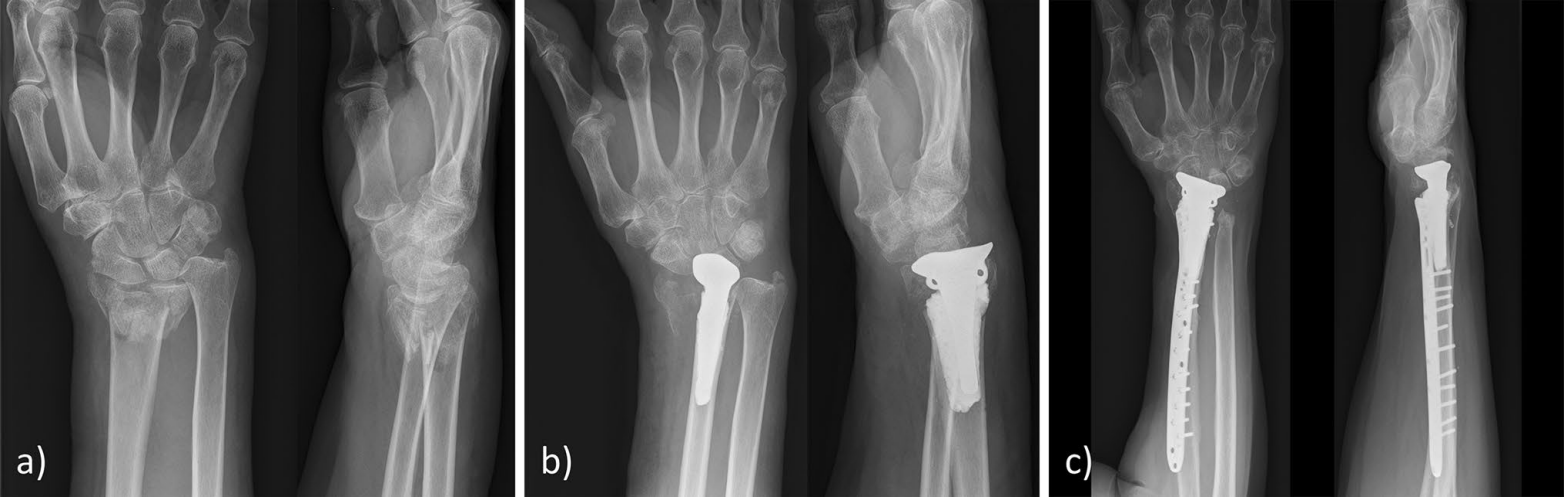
a Radiograph of the initial fracture. b Radiocarpal dislocation after hemiarthroplasty from a palmar approach. Revision included derotational osteotomy with a Darrach procedure and a pronator quadratus interposition transfer. c Radiograph at the follow-up 16 months after revision
